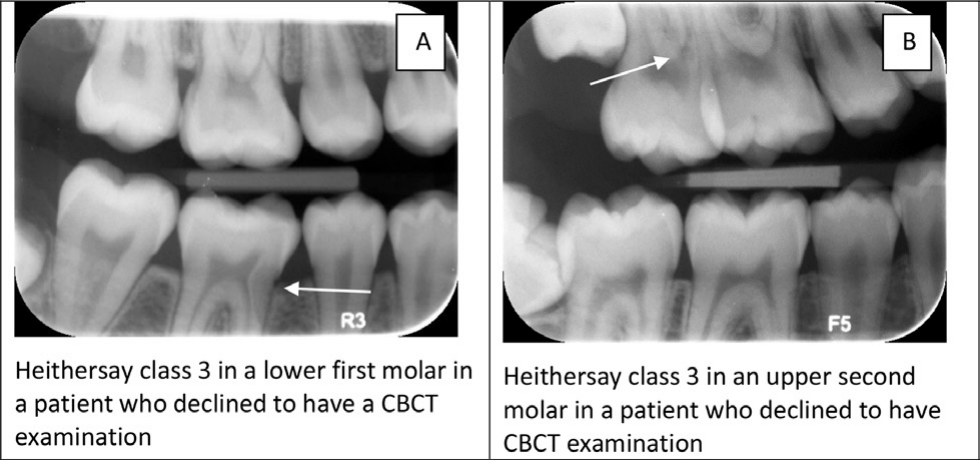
Examples of teeth suspected with ECR where the patient declined a CBCT examination. (A) ECR is suspected in the lower first molar (arrow); (B) ECR is suspected in the upper second molar (arrow). CBCT, cone beam CT; ECR, external cervical resorption.
